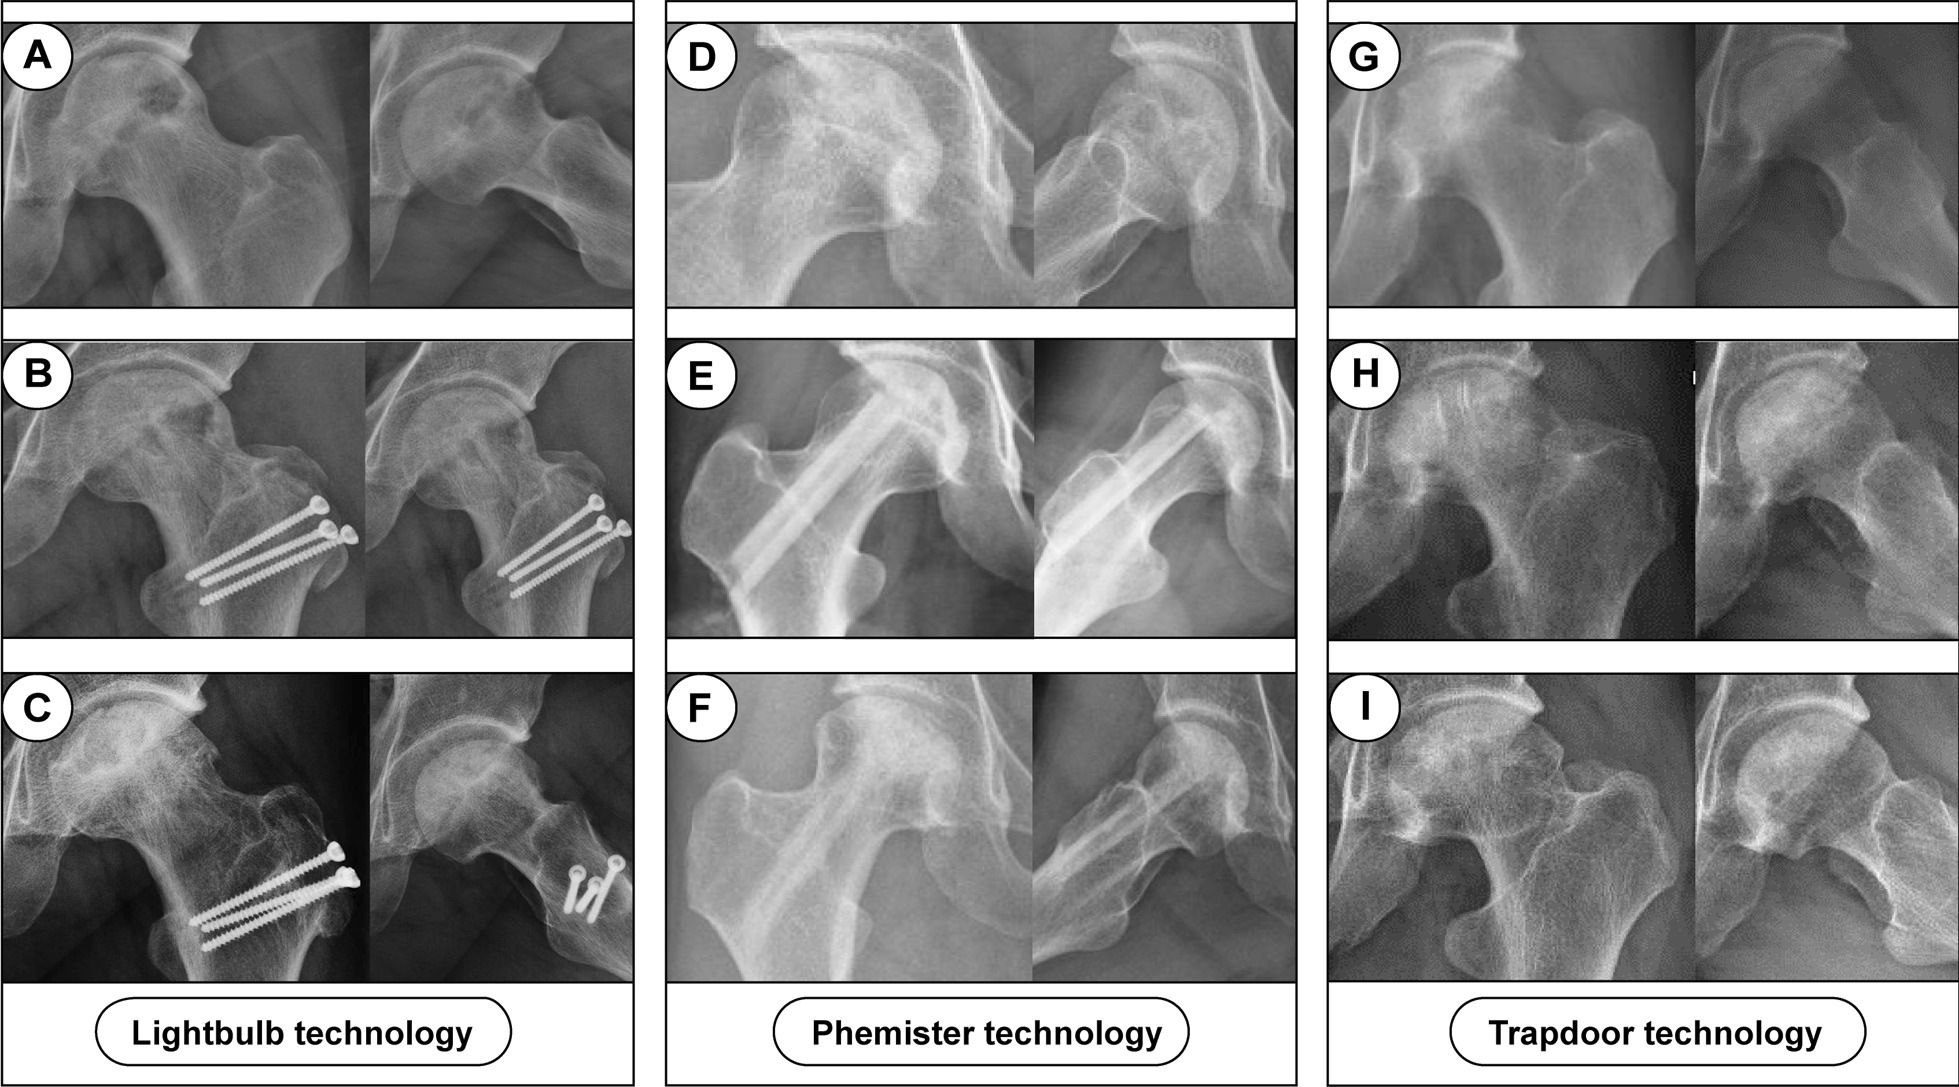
Three patients with ONFH underwent NVBG with three surgery techniques. A–C A 33-year-old male patient left alcohol-induced ONFH; B postoperative radiograph showing that the left femoral head was underwent NVBG with lightbulb technique, and the frog lateral x-ray position was not standard because of pain; C radiographs taken 30 months post operation show a restoration of bone. D–F A 24-year-old female patient right steroid-induced ONFH; E postoperative radiograph showing that the right femoral head was underwent NVBG with Phemister technique; F radiographs taken 75 months post operation show no further collapse happened; G–I A 41-year-old male patient left steroid-induced ONFH; H postoperative radiograph showing that the left femoral head was underwent NVBG with trapdoor technique; I radiographs taken 63 months post operation show the necrotic area was partially repaired
